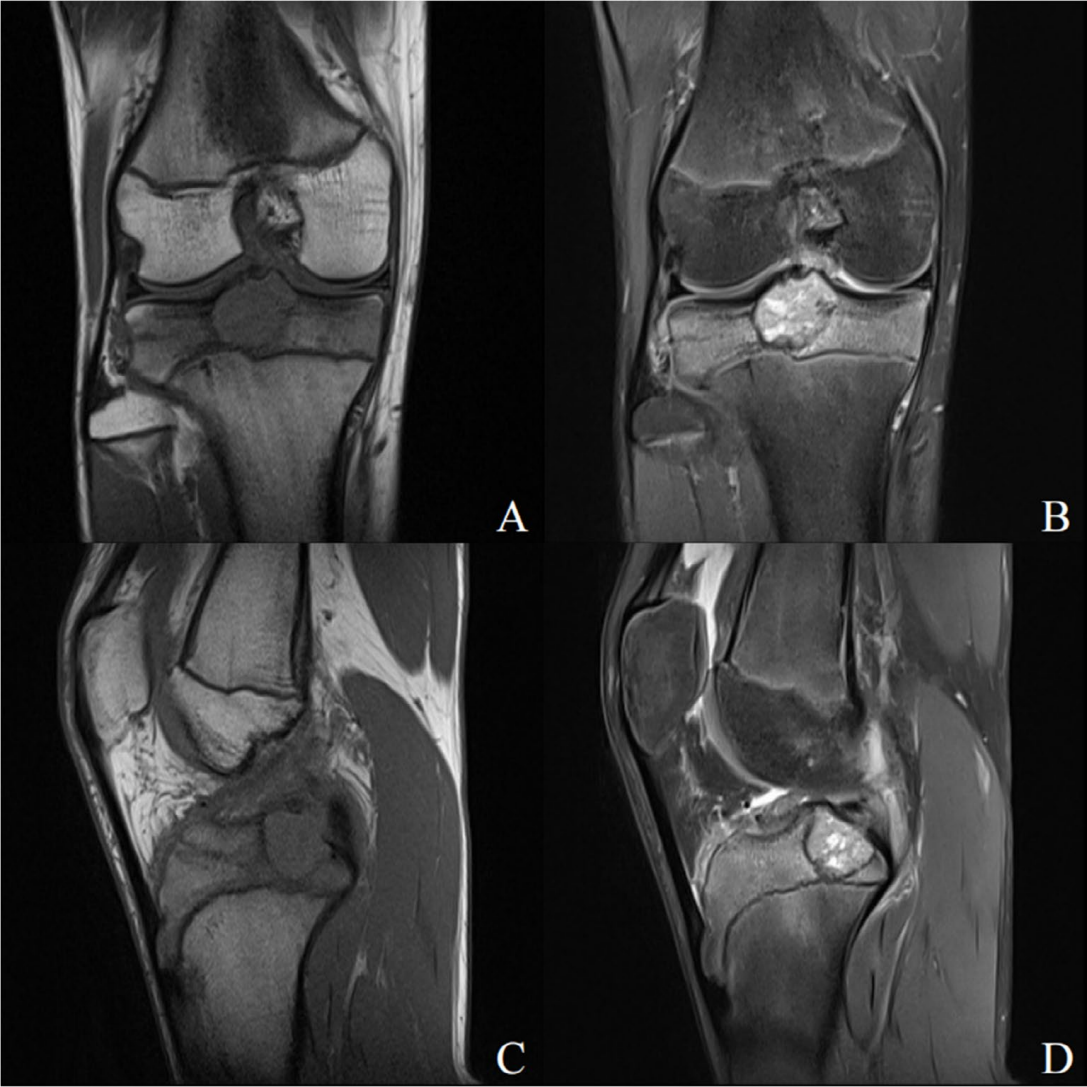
Magnetic resonance images of proximal tibial lesion in right knee: coronal T1‐weighted image (A), coronal T2‐weighted image view (B), sagittal T1‐weighted image (C), and sagittal T2‐weighted image (D). A well‐circumscribed septate lesion is seen, consisting of lobules with evidence of neighboring marrow edema.
